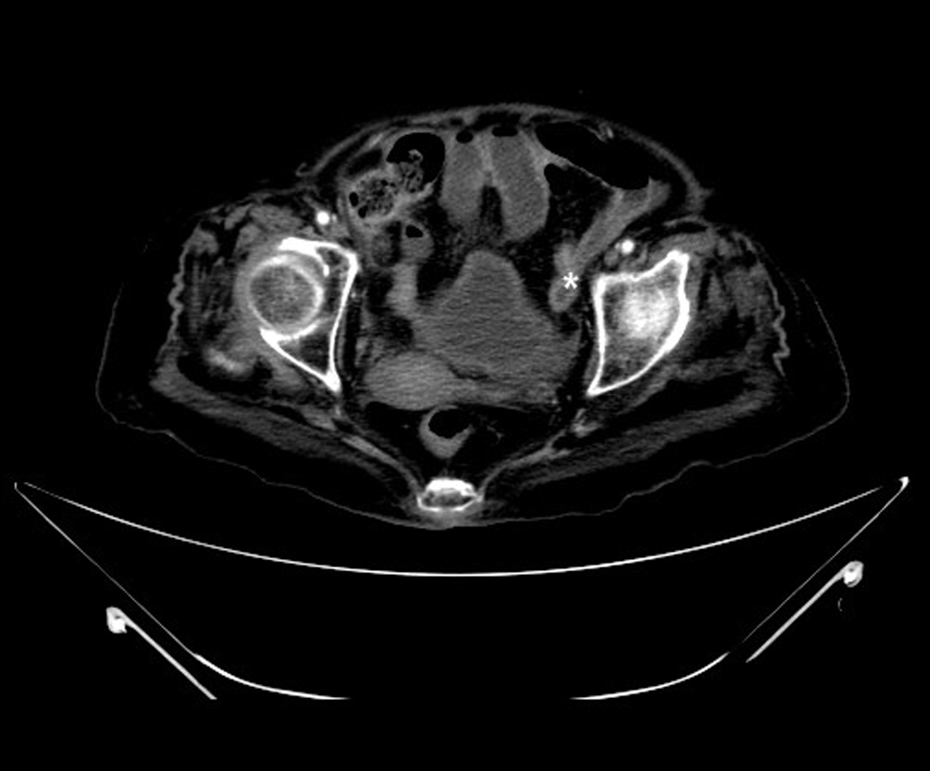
Abdominal computed tomography. Coronal view reveals a transitional region (asterisk) at the left lower abdomen.
